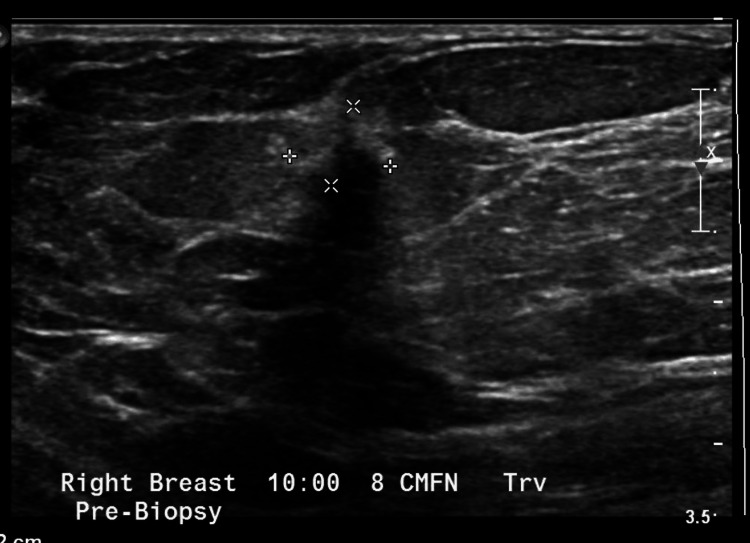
Ultrasound of the right breast. Ultrasound of the right breast further characterized the 10 o'clock lesion that was biopsy-proven to be invasive lobular carcinoma. The borders of the lesion are defined by "x".
